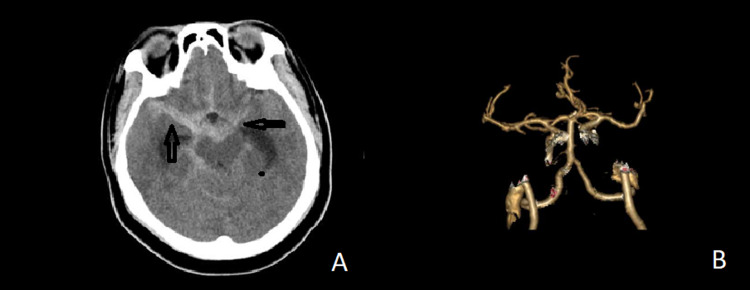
(A) Axial slide showing subarachnoid bleed with intra-ventricular extension and acute obstructive hydrocephalus. (B) CT angiography showing no vascular abnormality in the posterior circulation.
